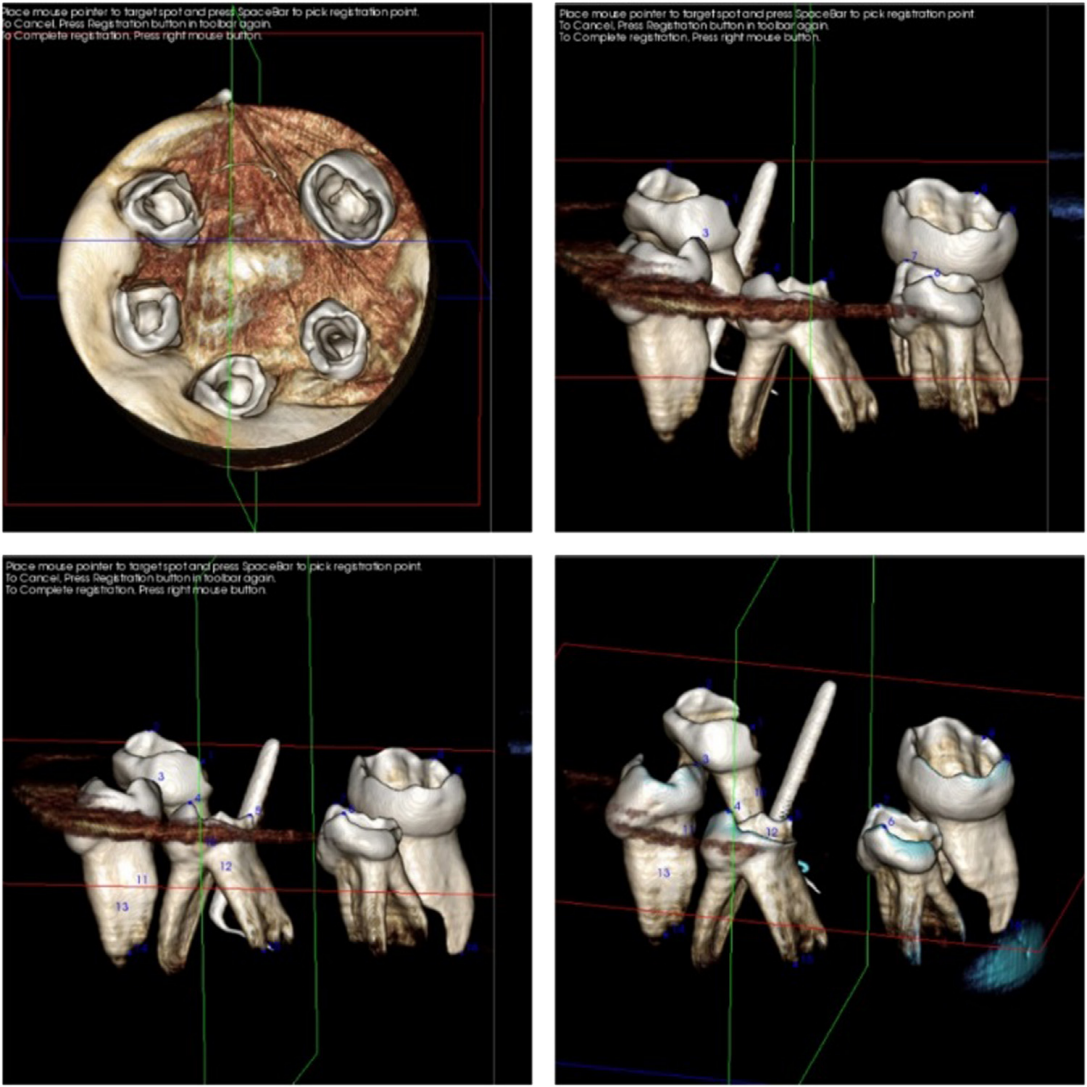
Photograph showing cone-beam computed tomography (CBCT) superimposition that ensures standardisation of pre- and post-CBCT scanning.
